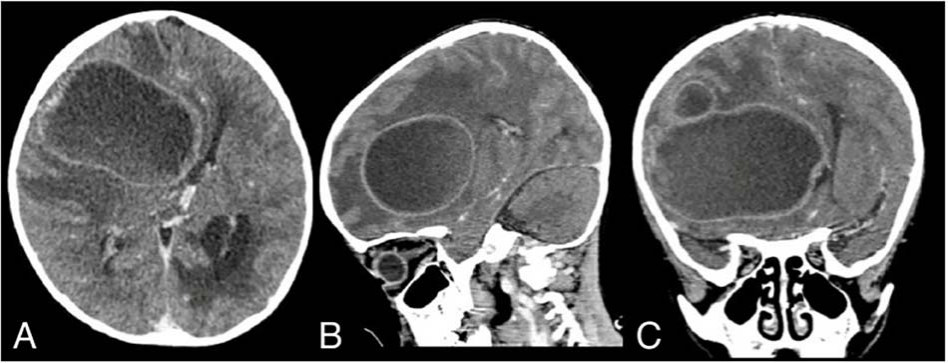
Admission contrast head CT scan showing a large hypodense lesion with ring-enhancement on the right frontotemporoparietal lobe; (A) axial view; (B) sagittal view; (C) coronal view.
